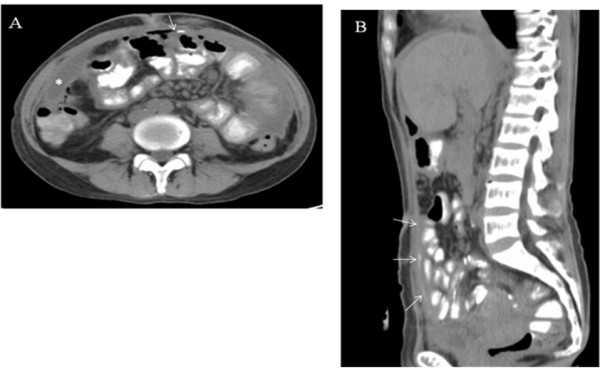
A and B. Repeated CT scan with patient ingesting 200 ml of Ultravist® medium. Both transverse (A) and sagittal (B) sections showed suspicious medium leak (arrow) but the amount was small and inconclusive to reveal the site of leakage. The pus (*) had been partially drained.
